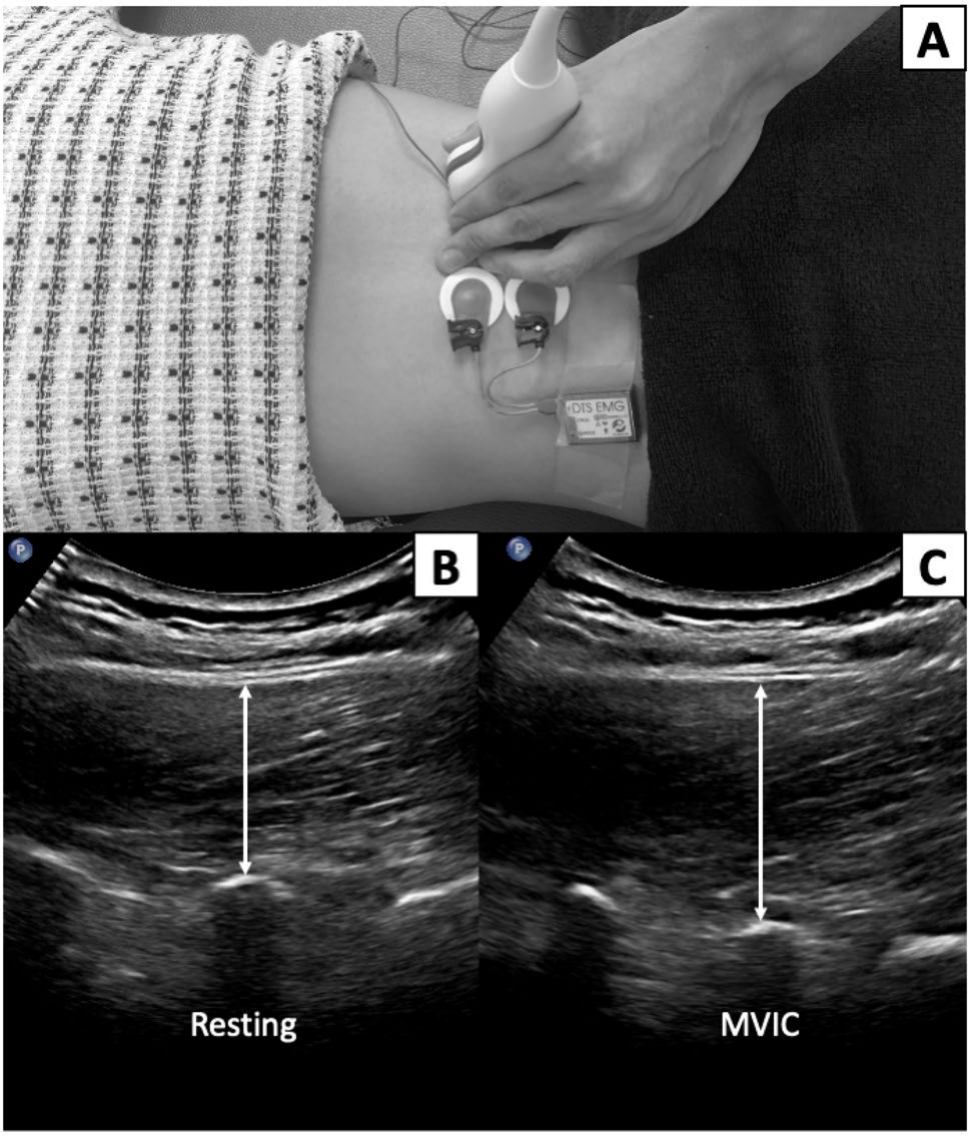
Example of rehabilitative ultrasound imaging protocol to measure right lumbar multifidus muscle (A) and muscle thickness during resting (B) and maximum voluntary isometric contraction (MVIC; C) derived from the distance between tip of L4-5 facet joint and lower border of thoracolumbar fascia.
